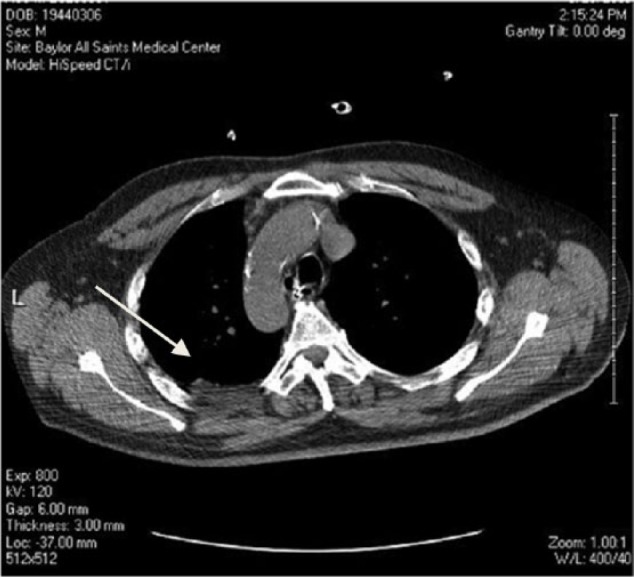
June 2008, CT scan showing a 1-cm noncalcified nodule within the upper lobe
